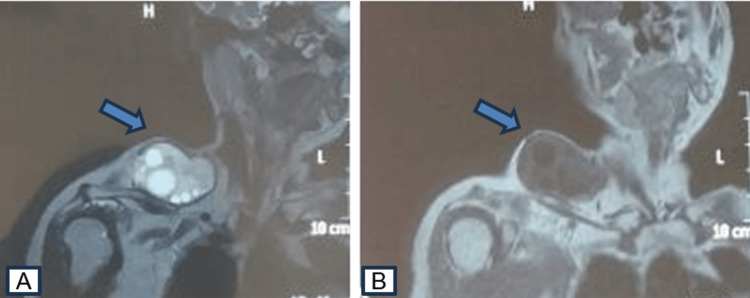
MRI confirming the multilocular appearance of the mass (A, B). MRI: magnetic resonance imaging
